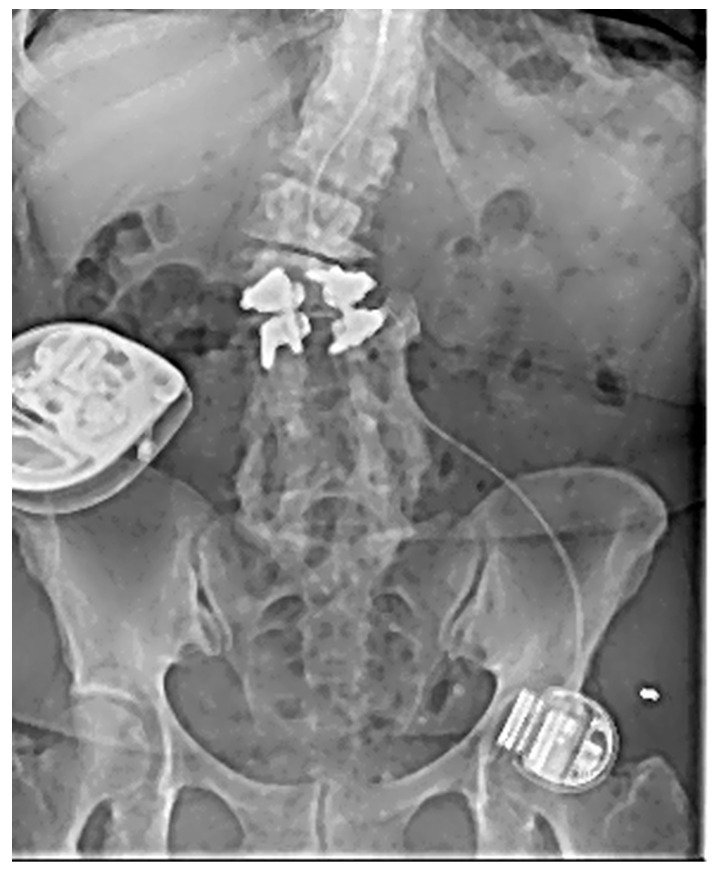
Example of an X-ray of a patient with combined therapy (SCS + IDD).
